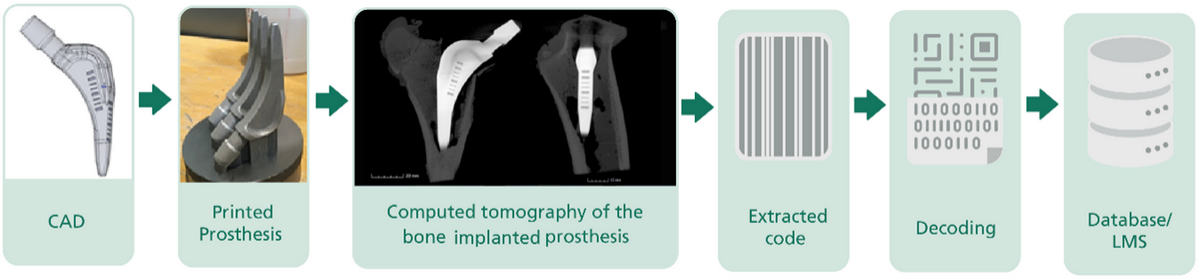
Example of the component (part) identification using a Pharmacode integrated into the hip prosthesis and data extraction from the identifier in the implanted state by computed tomography. CAD: computer-aided design; LMS: laboratory management system.
